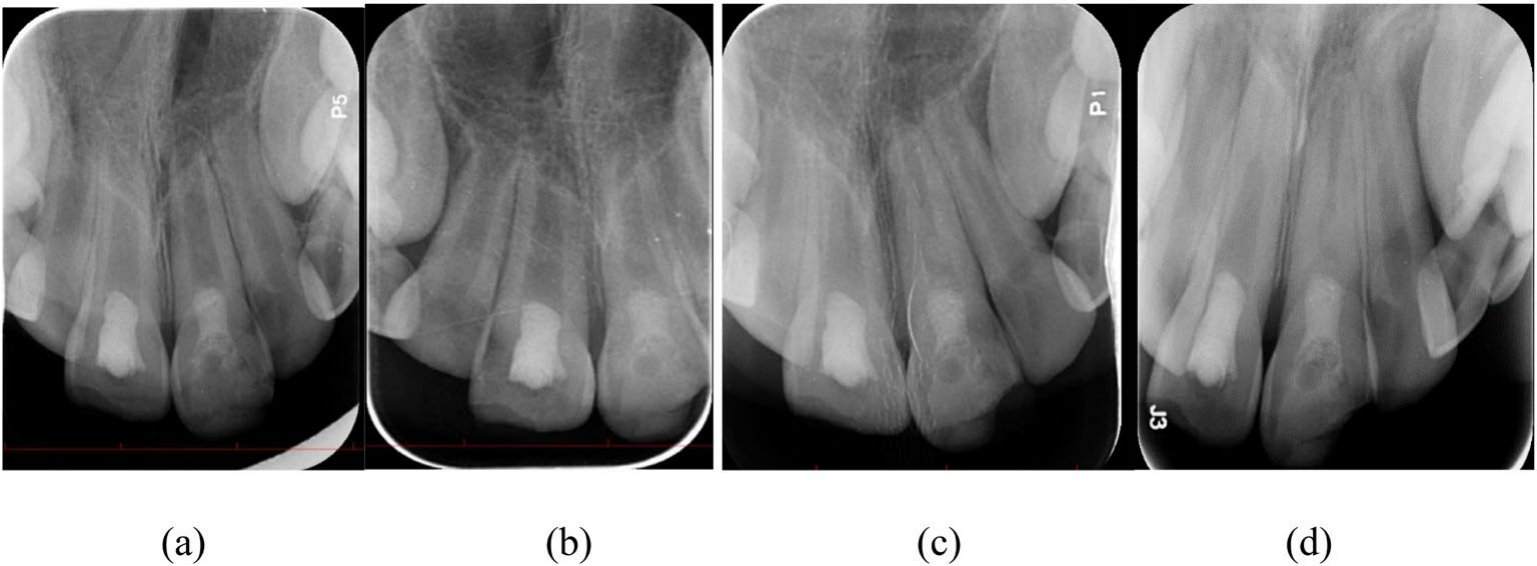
Follow‐up radiographs at (a) 3 months, (b) 6 months, (c) 12 months, and (d) 24 months.
